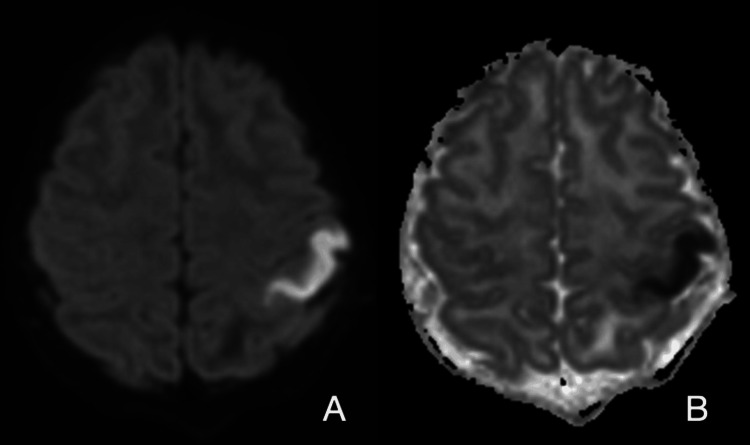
Brain MRI of acute ischemic stroke. (A) Diffusion-weighted imaging (DWI) showing restricted diffusion in the left postcentral gyrus. (B) Corresponding apparent diffusion coefficient (ADC) map demonstrating low signal, confirming acute ischemic stroke.
